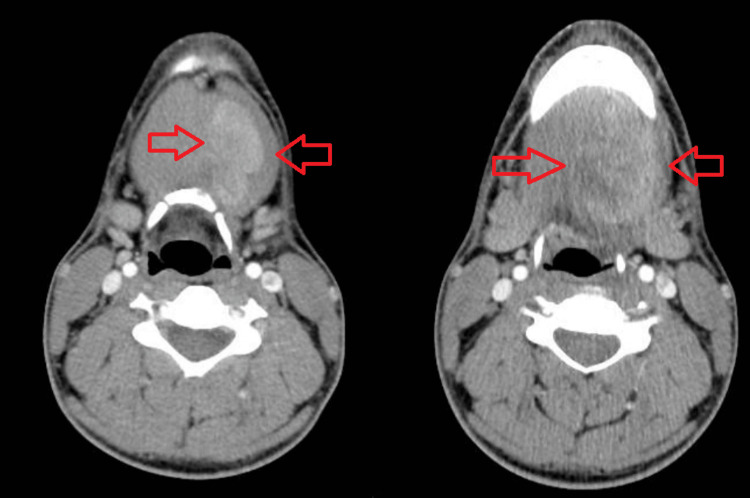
CT scan of the neck with intravenous contrast enhancement. Two different radiographic cuts: the higher cut and the lower cut, progressing from right to left.
